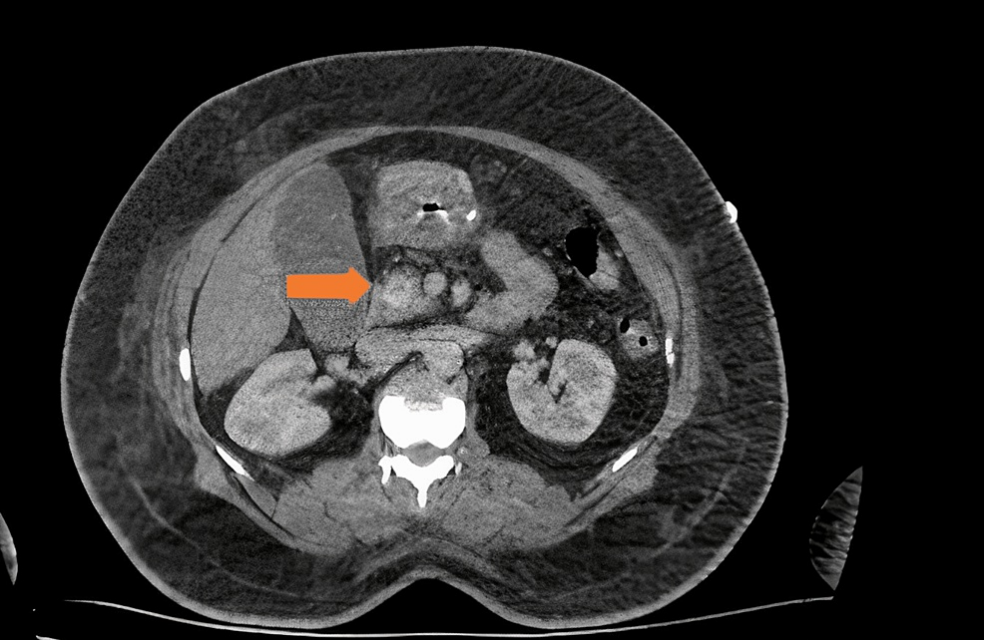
A CT of the abdomen showed a solitary nodule in the pancreas.
